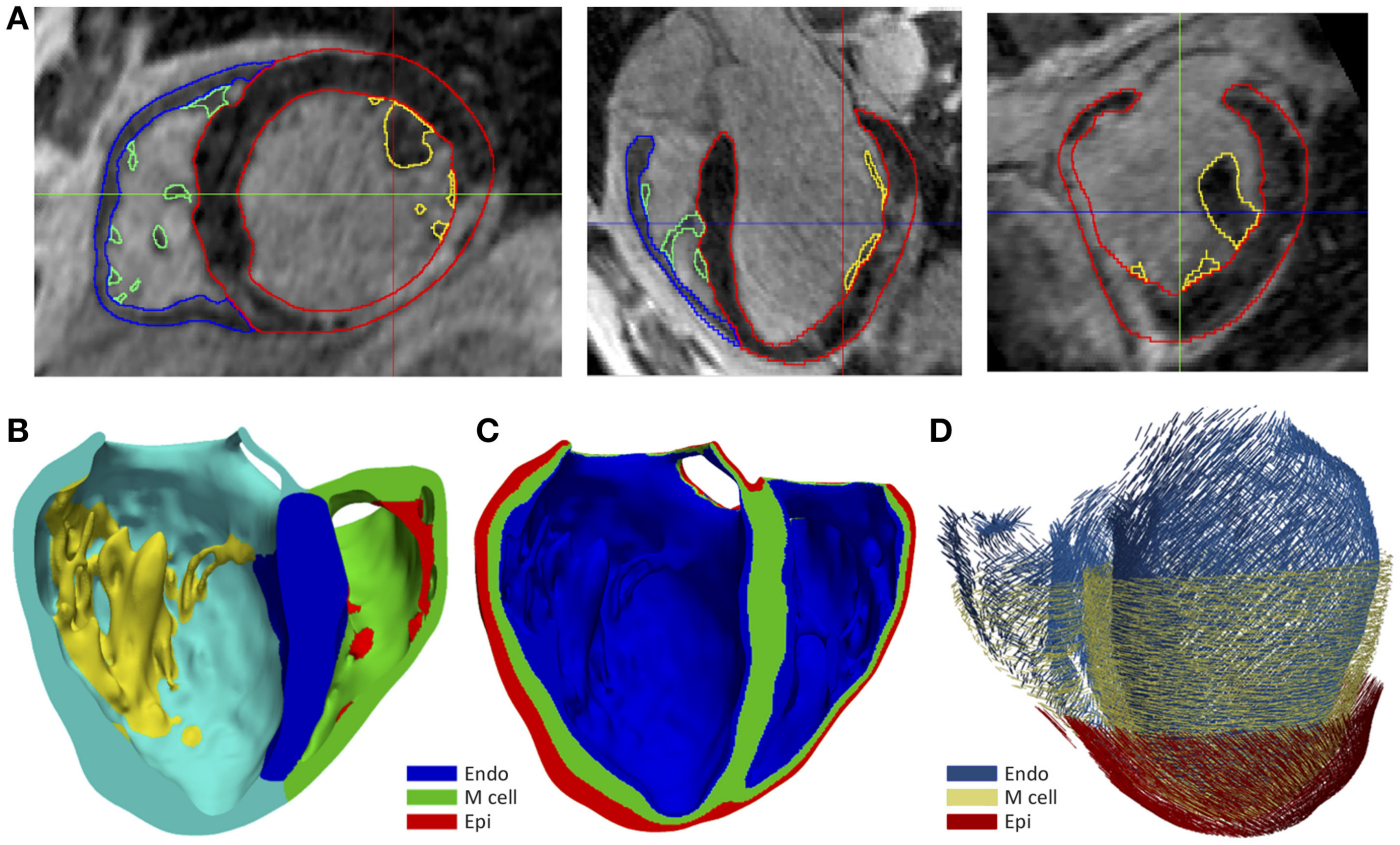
(A) Manual segmentation process of cardiac DE-MRI performed with Seg3D, displaying three slices corresponding to the standard cardiac planes: short-axis (*left*), four-chamber plane (*center*) and long-axis showing the LV (*right*). (Note that in those views the LV appears on the right side and the RV on the left). Contours highlighted in distinct colors show: LV myocardium including the septum (*red*), RV myocardium (*blue*) and papillary muscles and endocardial trabeculations of LV (*yellow*) and RV (*green*). (B) Posterior view of a coronal cross-section (four-chamber plane) of the hexahedra-based FEM volume mesh of the 3D *patient-specific* ventricular model. Various cardiac regions are labeled with different colors: septum (*blue*), LV free wall (*cyan*), RV free wall (*green*) and papillary muscles and main endocardial trabeculations of LV (*yellow*) and RV (*red*). (C) Transmural heterogeneity of ventricular myocardium, showing the distribution of the three different kinds of ventricular myocytes: endo-, mid- (M cells), and epicardial cells. (D) Left antero-lateral view of the 3D ventricular model displaying a representation of cardiac fibers orientation at different wall depths (endocardium, mid-myocardium, and epicardium).
